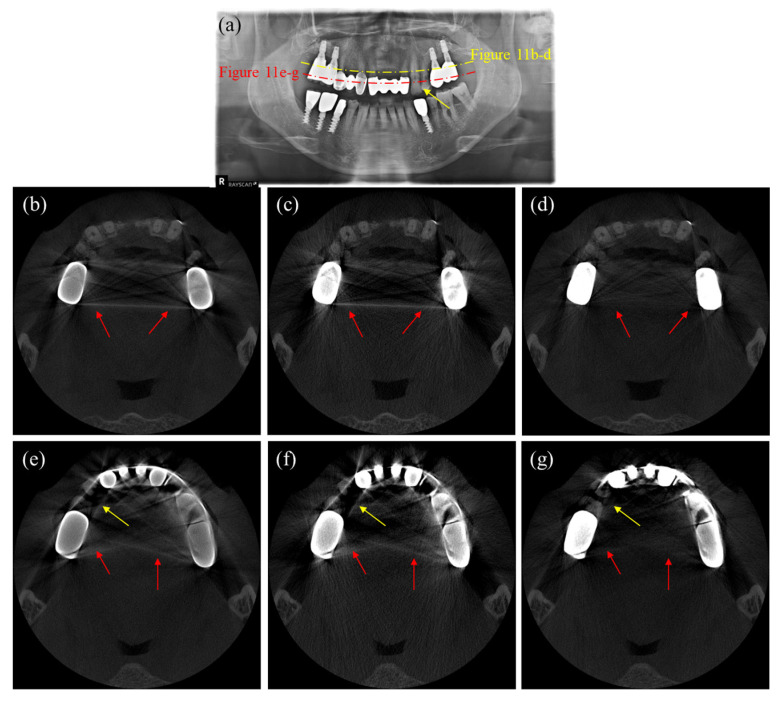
Images of a patient having multiple dental implants and crowns. (a) The panoramic X-ray image, (b,e) the uncorrected CT images, (c,f) the CT images corrected by the DSC method, and (d,g) the CT images corrected by the proposed method. (Contrast window is [−1117~8564] in Hounsfield Unit).
